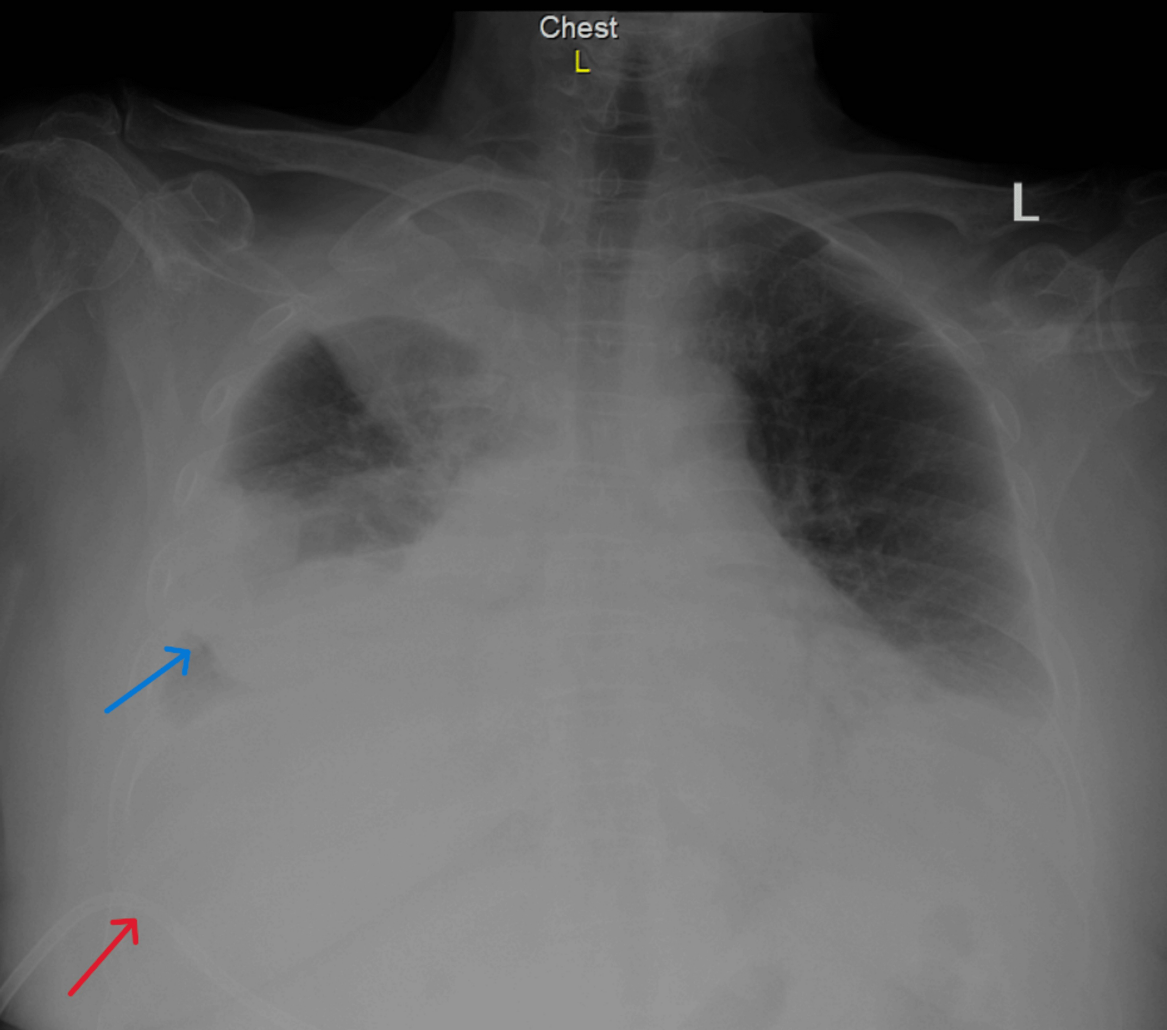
Chest radiograph showing right-sided loculated pleural effusion Blue arrow: right-sided loculated pleural effusion; Red arrow: Percutaneous transhepatic drain
